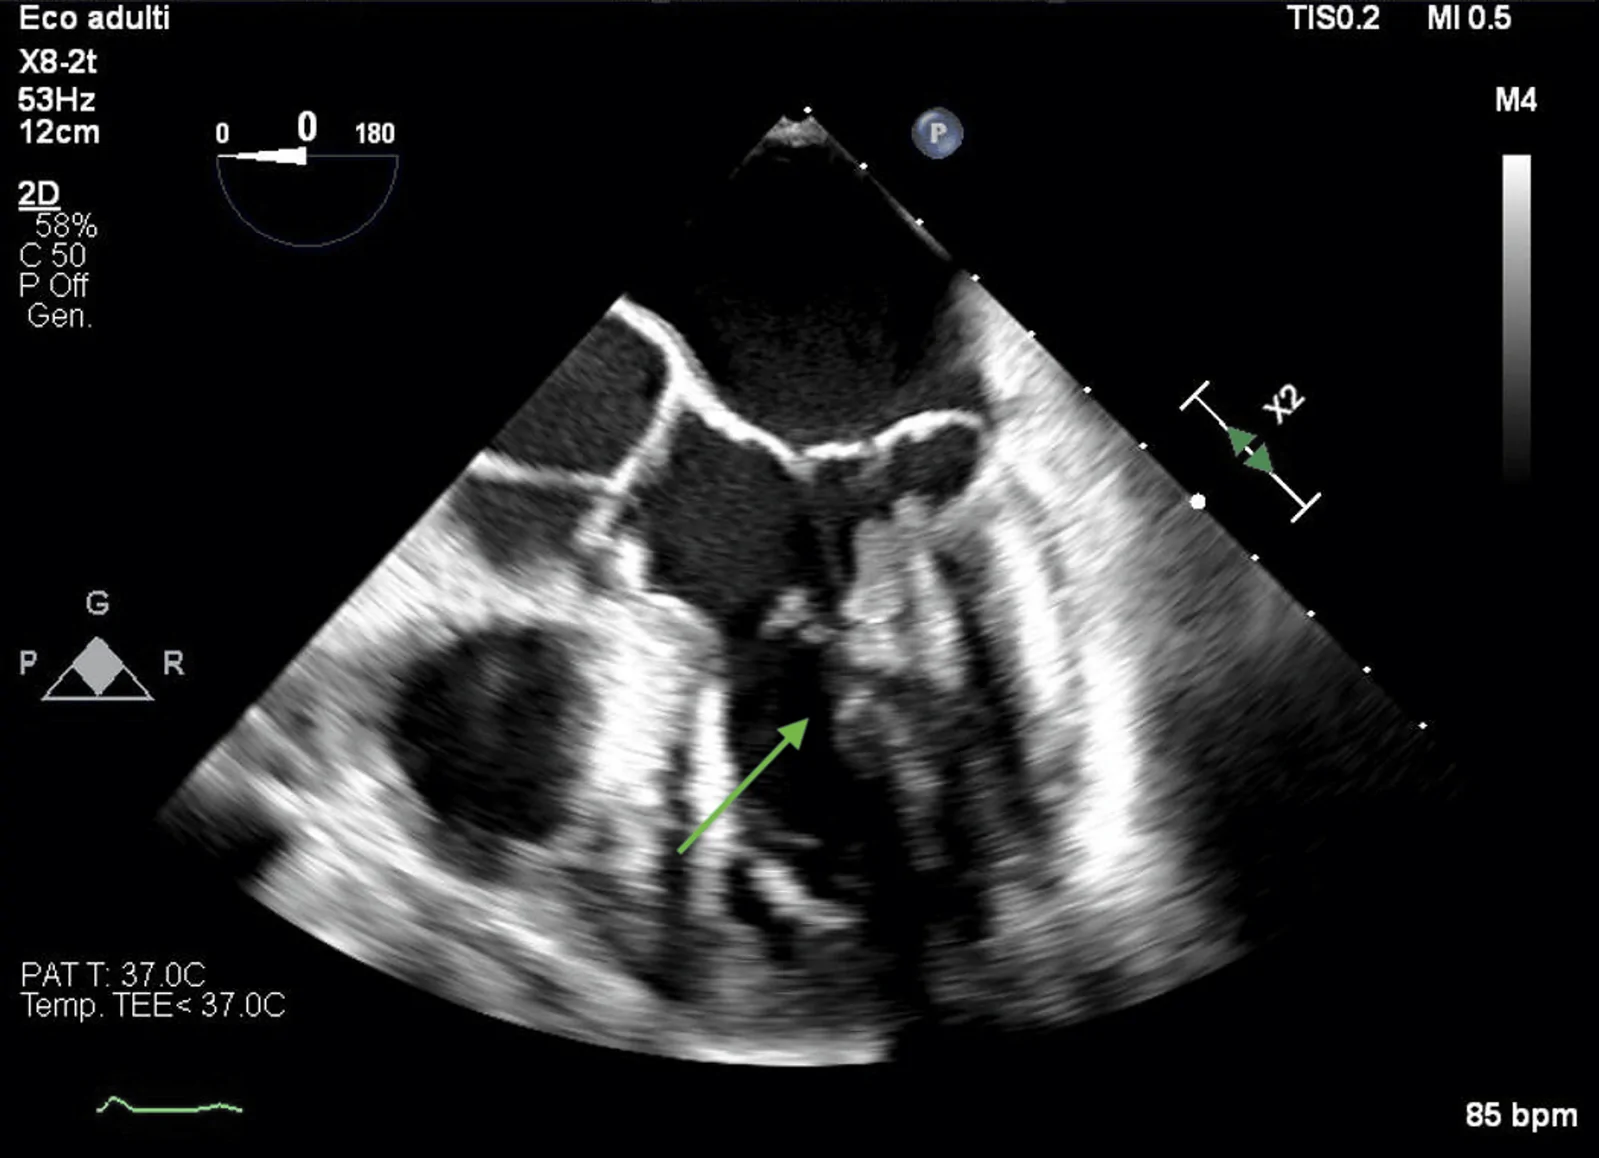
TEE showing mitral endocarditis as initially undetermined mass in the left ventricle TEE: transesophageal echocardiogram
